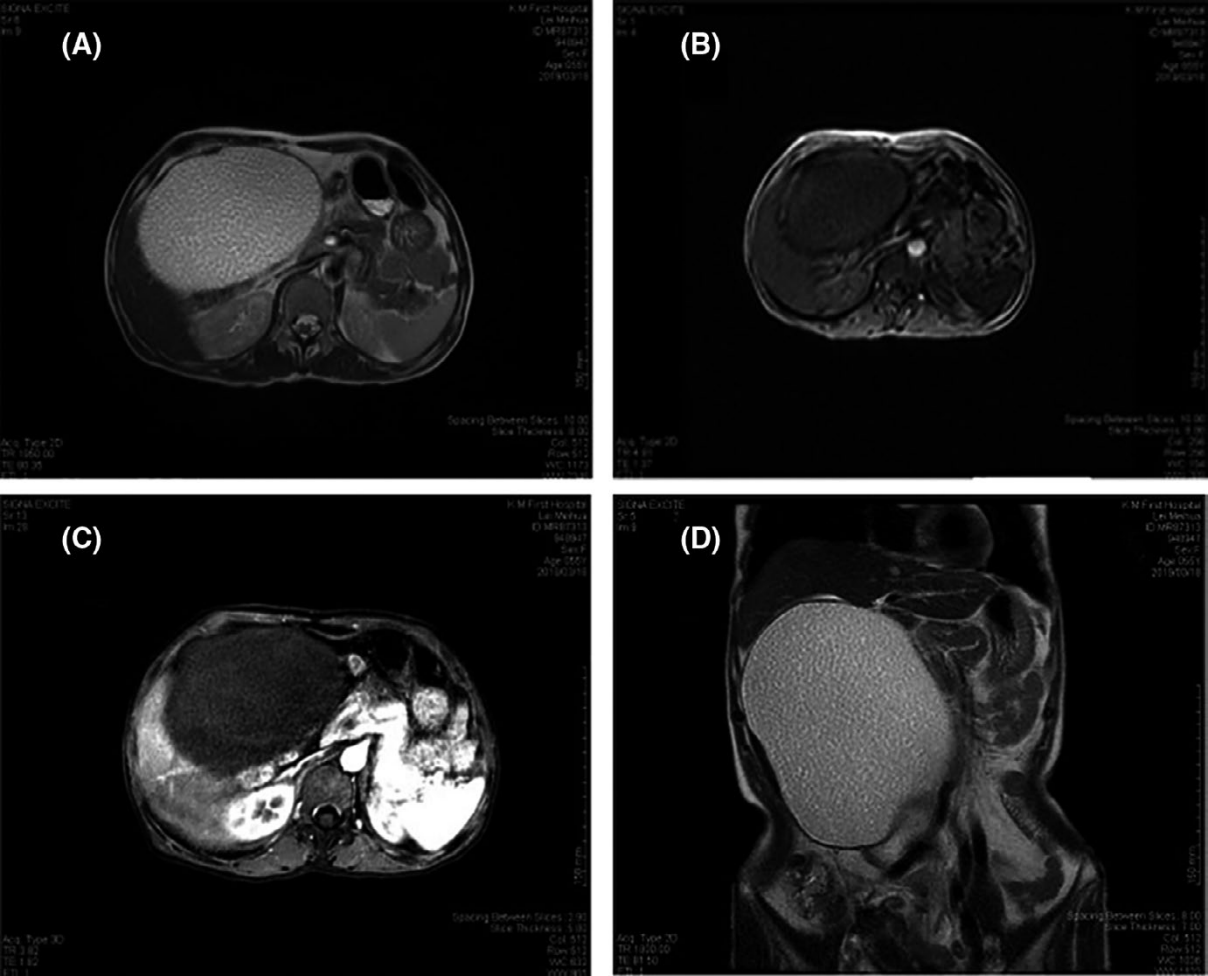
MRI results of the patient. A, T1WI: giant mass‐like high signal shadow with clear boundaries. B, T1 inversion: low signal shadow with clear boundaries. C, Enhancement: no obvious enhancement. D, T2WI coronal view: high signal shadow with obvious compression of adjacent tissues. Summary: A large mass with long T1 and long T2 signals was observed in the right abdominal cavity, with a smooth edge and clear boundaries; the size was approximately 10.3 × 14.2 × 18.3 cm. There was no significant enhancement at the edge in enhanced imaging
